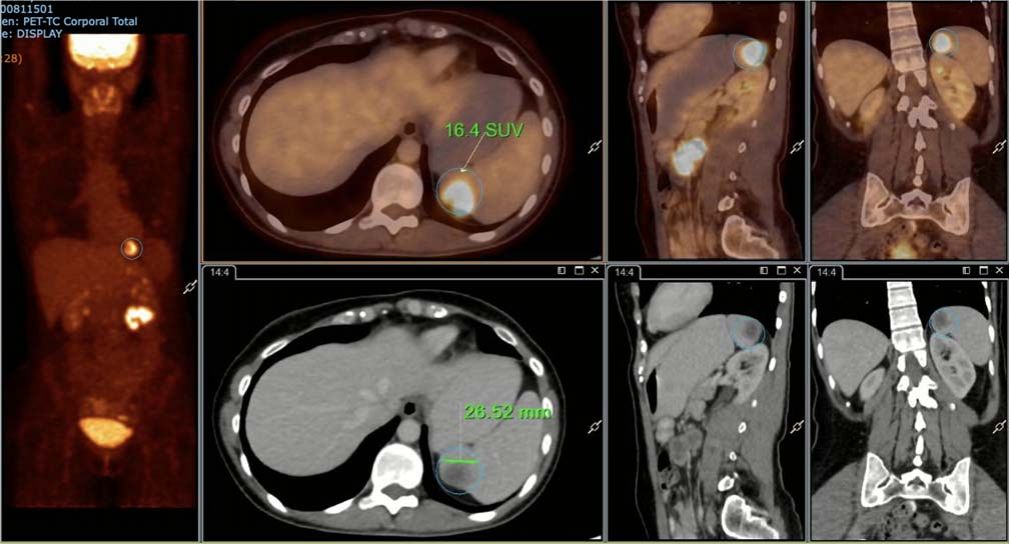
PET/CT showing a hypermetabolic splenic nodule in the upper pole (SUVmax 16.4).
